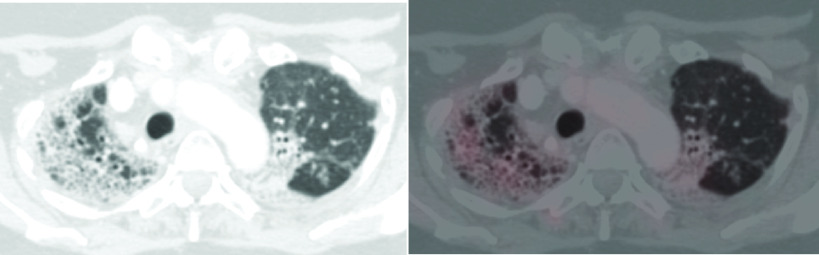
Post treatment images of patient no. 14, case of leiomyosarcoma. (A) Maximum intensity projection image of fused fluorodeoxyglucose positron emission tomography with computed tomogram (FDG PET-CT) of patient no. 14 showing decreased areas of tracer uptake. (B) Resolution of lytic skeletal lesions in axial contrast-enhanced computed tomography images. (C) No FDG uptake in fused PET-CT. (D) Lung metastases. (E) Mild FDG uptake in fused PET-CT. (F) Resolution of intramuscular deposits. (G) No FDG uptake in fused PET-CT image. Overall findings suggestive of partial response.
